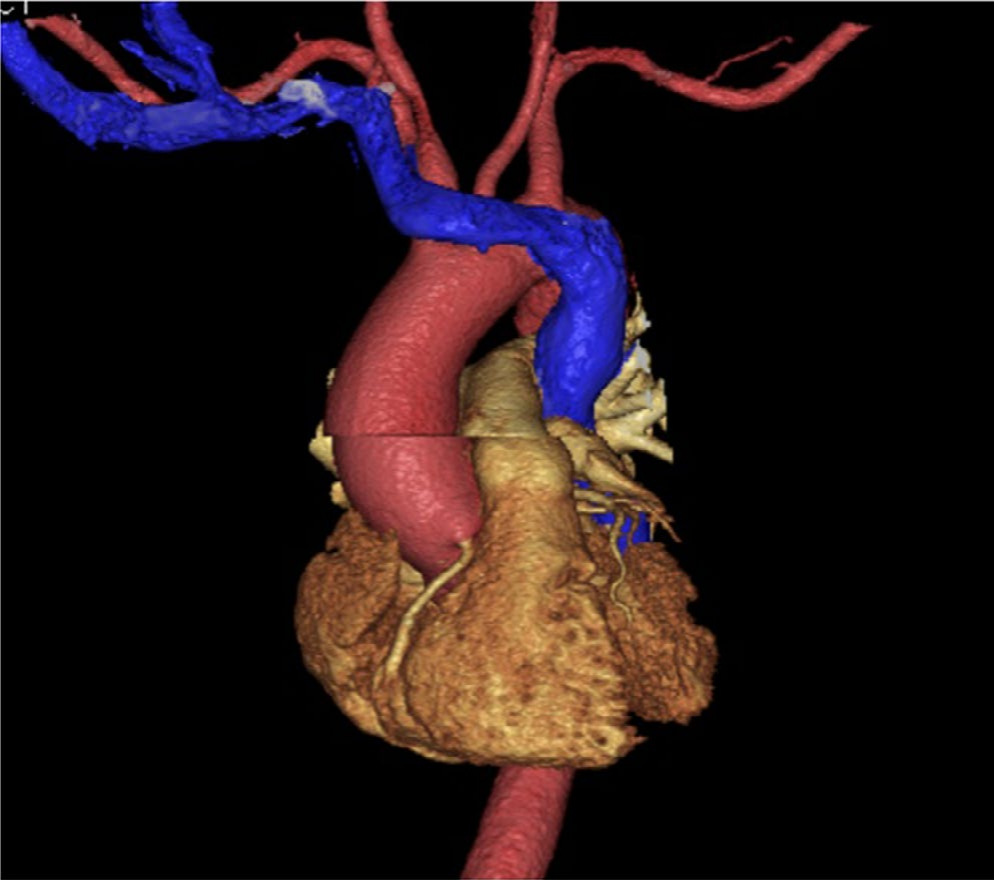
A three‐dimensional CT image (anterior view): A persistent left superior vena cava without a superior vena cava on the right. CT, computed tomography
